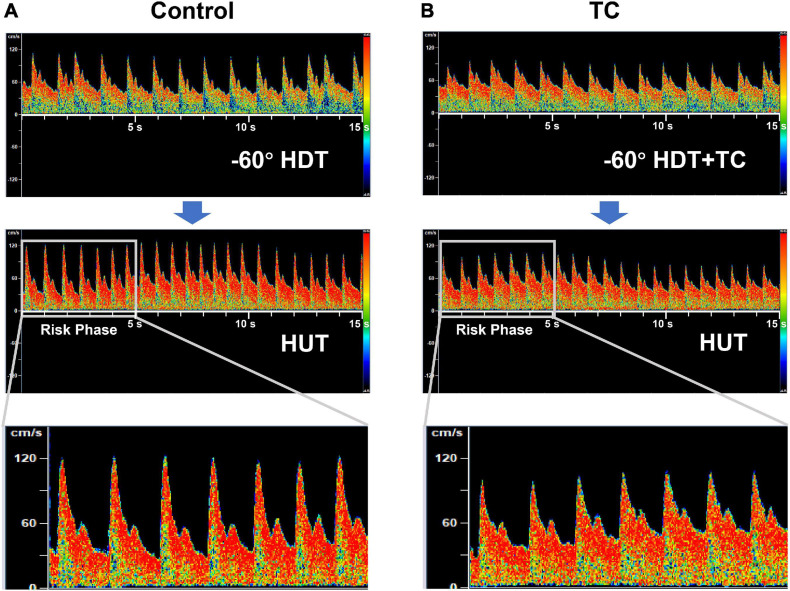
Representative transcranial Doppler records of cerebral blood flow velocity during the rapid HDT-to-HUT transition in control (A) and TC (B) bouts. The duration of each record in the top and middle panel is 15 s. The first 5-s records of cerebral blood flow velocity during HUT after the rapid transition were zoomed in the bottom panel, as the loss of consciousness in pilots during PPM generally happens just after the rapid transition from −Gz to + Gz (risk phase). HDT, head down tilt; HUT, head up tilt; TC, thigh cuff; PPM, push—pull maneuver.
